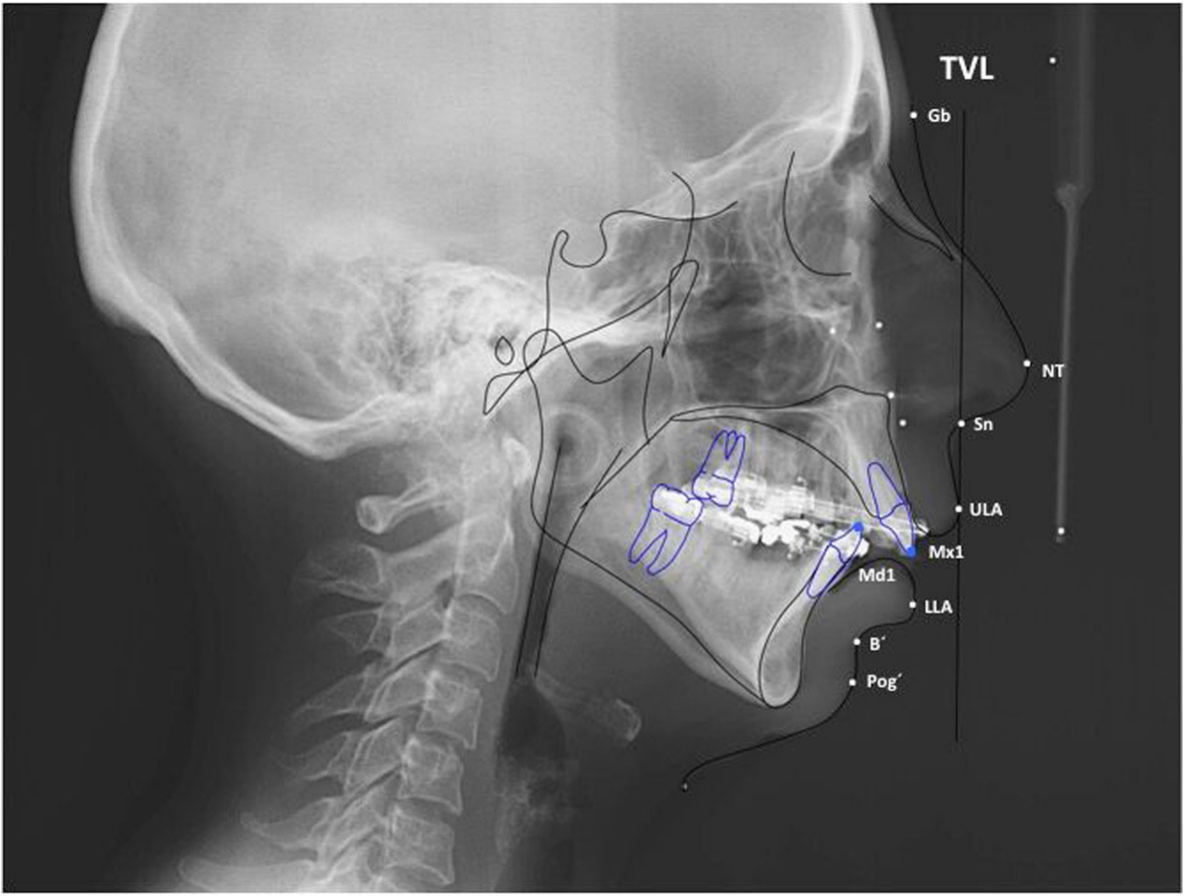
Sample of a cephamolmetric radiograph of the lateral soft tissue facial analysis
